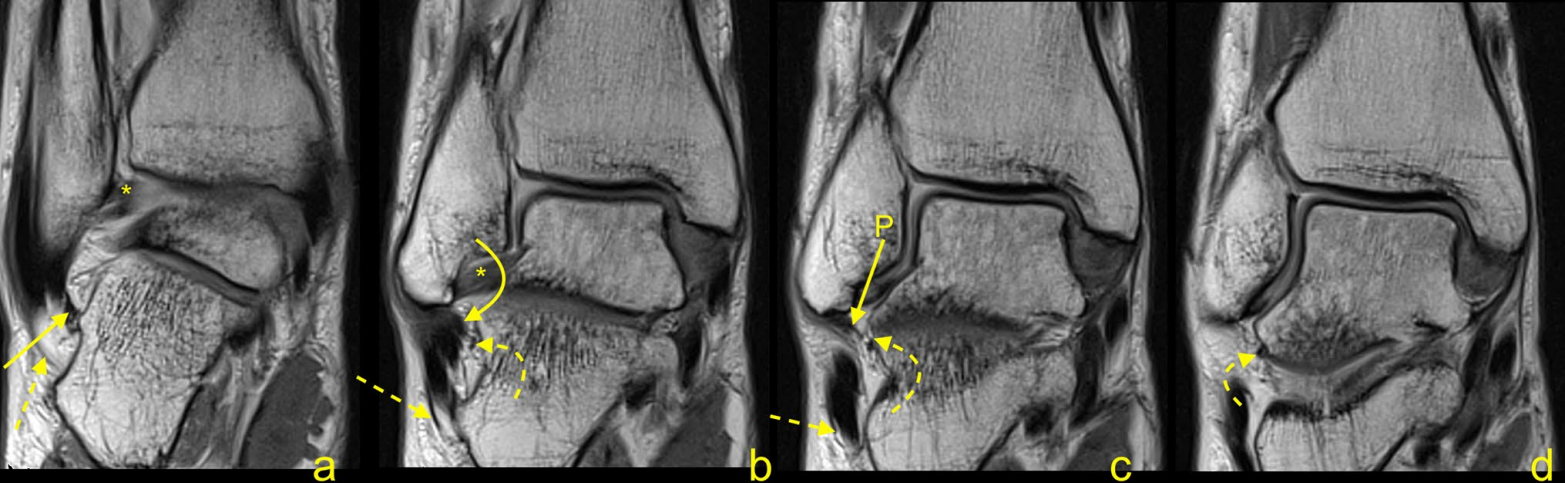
A 41-yeard-old patient with suspicion of a stress fracture of the distal tibia. No fractures were revealed on the MRI. The variably occurring accessory fascicle of the CFL inserts on the talus (curved dashed arrow). The CFL (straight arrow) in the distal part is not divided, and the proximal part (P) attaches to the lateral malleolus. The PTS (dashed arrow) and the PTFL (*).
